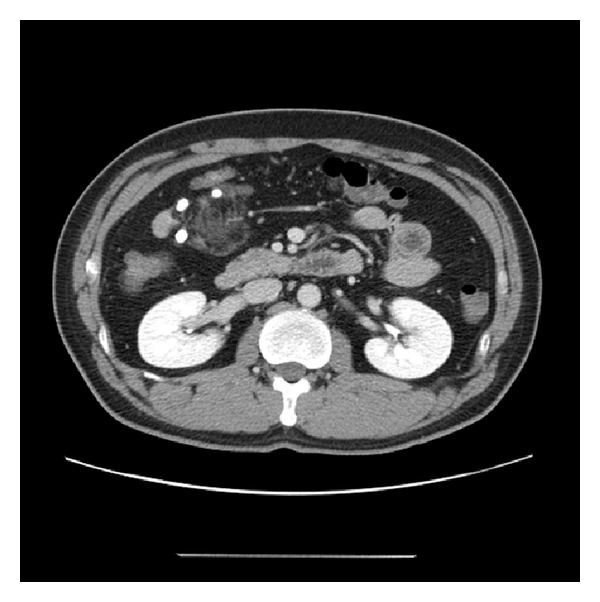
Axial contrast-enhanced CT scan. It shows the tumor measuring 4 cm in size and having fat component in the mesentery.
